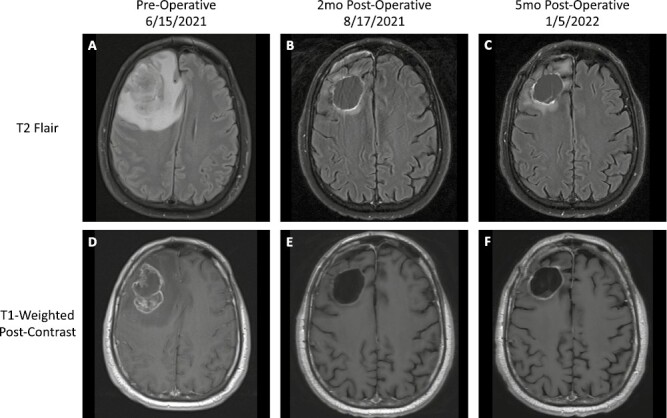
Disease progression within the brain. T2 FLAIR and T1-weighted postcontrast MRI scans showing the patient’s brain mass at presentation (A, D), 2 months following resection (B, E), and 5 months following resection (C, F).
